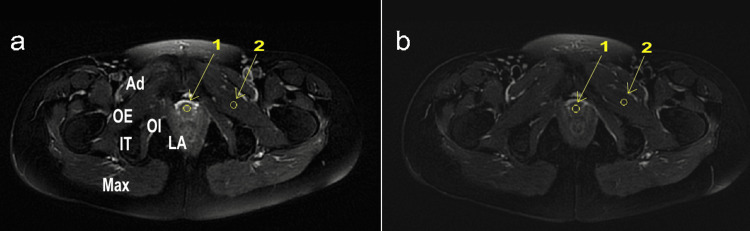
Changes in signal intensity ratio between the urethra and external sphincter on T2-weighted imaging a: before treatment; b: after treatment Ad: adductor muscles; OE: obturator externus muscle; IT: ischial tuberosity; OI: obturator internus muscle; LA: levator ani muscle; Max: gluteus maximus muscle The yellow circles represent the measurement of MRI values at the same location of the urethra (1) and OE (2).
